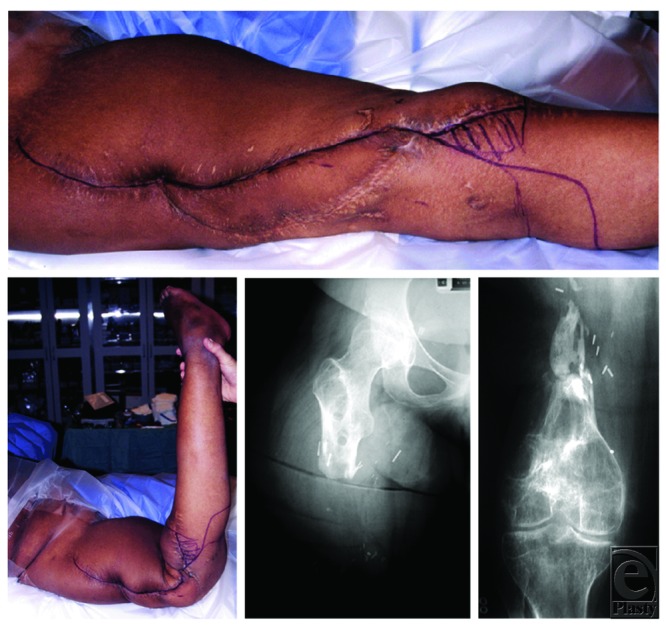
Clinical preoperative images. (*Top*) Extensive scarring. (*Bottom left*) An unstable thigh with 90° of dorsal extension in the distal thigh secondary to the lack of an intact femur. The thigh is freely mobile to deforming forces. Anteroposterior radiographs of the right hip (*bottom, middle*) and the right knee joint (*bottom, right*) display the major bony defect.
